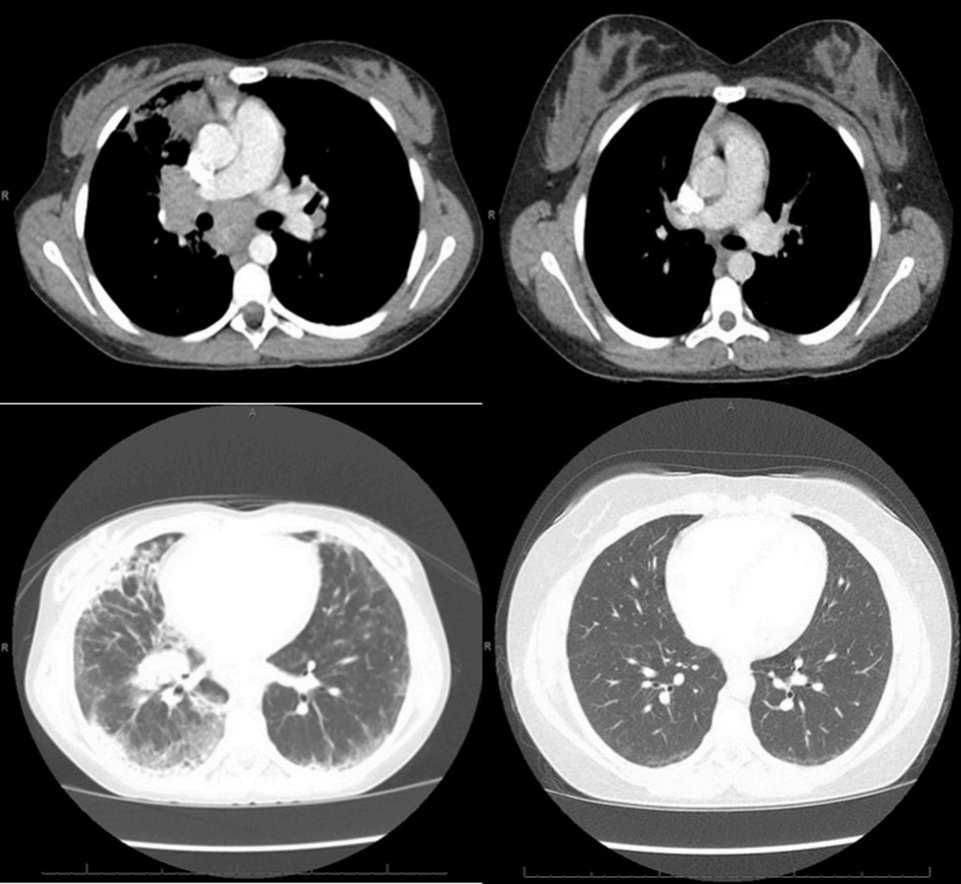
Left: CT of the chest obtained around the time of clinical presentation of GLILD, showing mediastinal and hilar lymphadenopathy, peripheral interlobular septal thickening, peripheral consolidation, and ground glass opacities, more prominent on the right lung than left. Right: CT of the chest obtained after treatment with 4 doses of rituximab and azathioprine showing resolution of the lymphadenopathy, consolidation and septal thickening and near resolution of the ground glass opacities.
